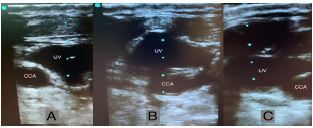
(A) Ultrasonography of patient’s left neck in a neutral position showing the common carotid artery (CCA) lateral to the internal jugular vein (IJV), (B) coursing posteriorly as it travels caudad in a rotational trajectory, and (C) ultimately reaching its typical orientation medial to the IJV.
